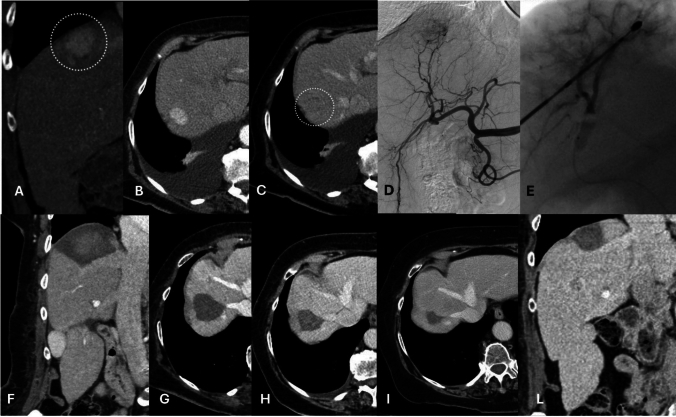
76 years old cirrhotic female, with a not capsulated 32 mm HCC in segment VII, hypervascular in the arterial phase (MIP 3 mm coronal reconstruction: a, and axial: b), with late wash-out c showed a (dotted circle), confirmed at the angiography (trans-femoral, anteroposterior projection digital subtracted angiography, d and treated e with percutaneous thermal segmentectomy. Post-procedural 24 h CT (venous phase coronal plane, f) showed a triangular necrotic area shape that encompassed all the HCC, thus configuring a complete response. The complete response was maintained at the subsequent follow-up, performed at one (g), twelve (h), twenty-four (i) and forty (l) months
